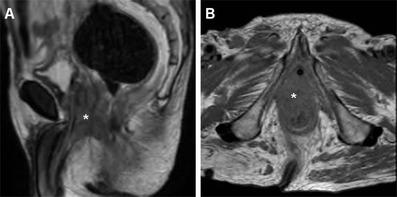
An elderly, 92-year-old man with previous prostatectomy and kidney failure has unenhanced MRI. Sagittal T2- (a) and axial T1-weighted (b) images show 5-cm long solid, inhomogeneous neoplastic tissue (*) extending from the anus to encase the proximal urethra (note catheter in place)
